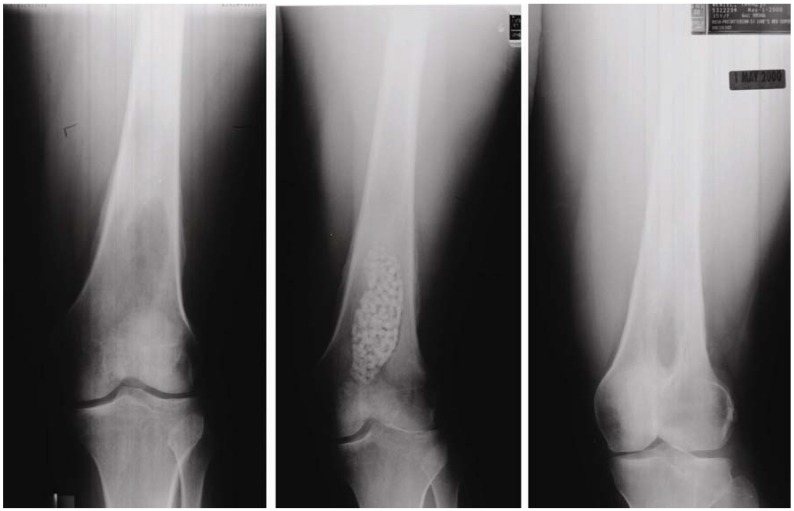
Radiographic assessment of OsteoSet(R) resorbable beads filled into the bone void of a 40-year-old female resulting from osteomyelitis, taken at: preoperation (left), postoperation (middle), and follow-up (right). (Photo courtesy of Wright Medical Technology).
